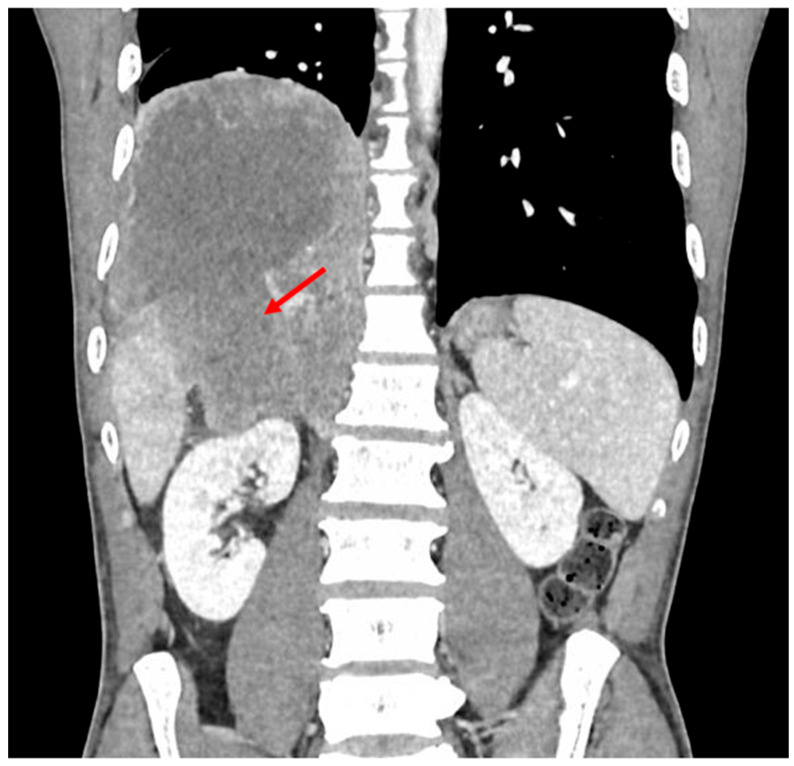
Contrast-enhanced abdominal CT scan (axial view) showing a large (15 cm) heterogeneous, necrotic adrenal mass with irregular contours, located bilaterally beneath the right diaphragmatic dome in the middle posterior region.
